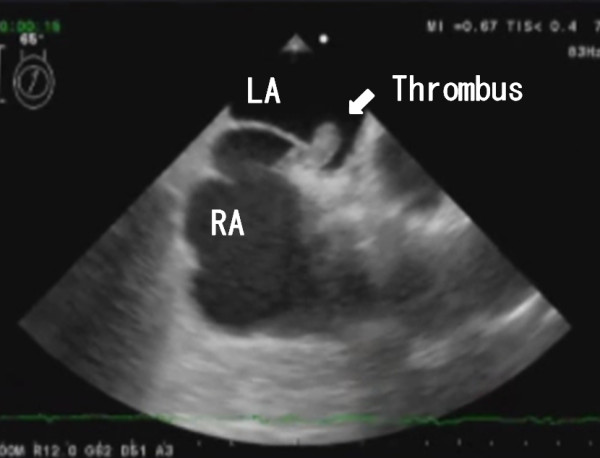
Transesophageal echocardiogram showing a thrombus entrapped in a patent foramen ovale.
